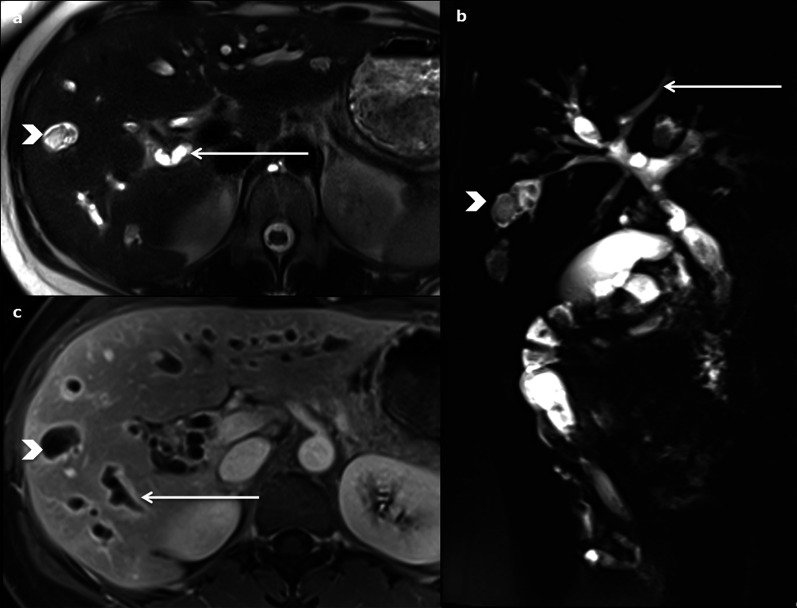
Caroli disease in a 24-year-old male. a Axial magnetic resonance imaging in T2 and (b) in T1 on portal venous phase show both fusiform (arrows) and connected round (arrowheads) dilatations, better depicted on magnetic resonance cholangiopancreatography images (c). There is no associated sign of fibrosis
